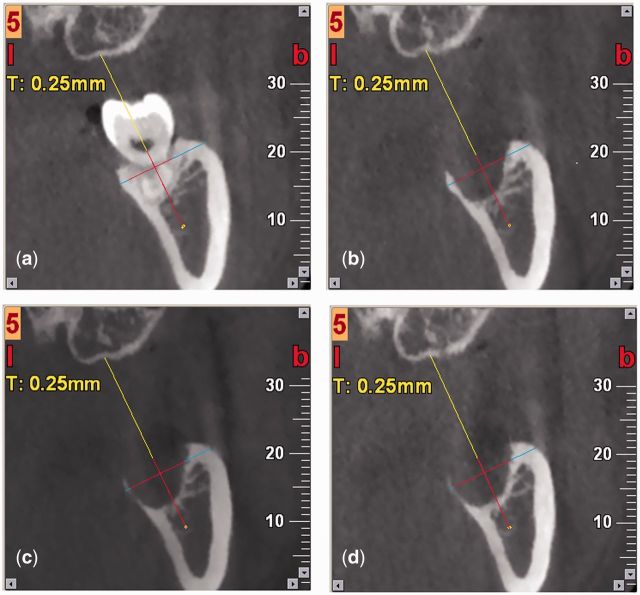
CBCT of experimental site for quantitative analysis of alveolar dimensional changes in horizontal and vertical directions. (a): before extraction; (b): 3 months after extraction; (c): 6 months after extraction; (d): 12 months after extraction. Reference line, lines for alveolar height and width, and lines for buccal and lingual bone wall thickness are marked separately.
